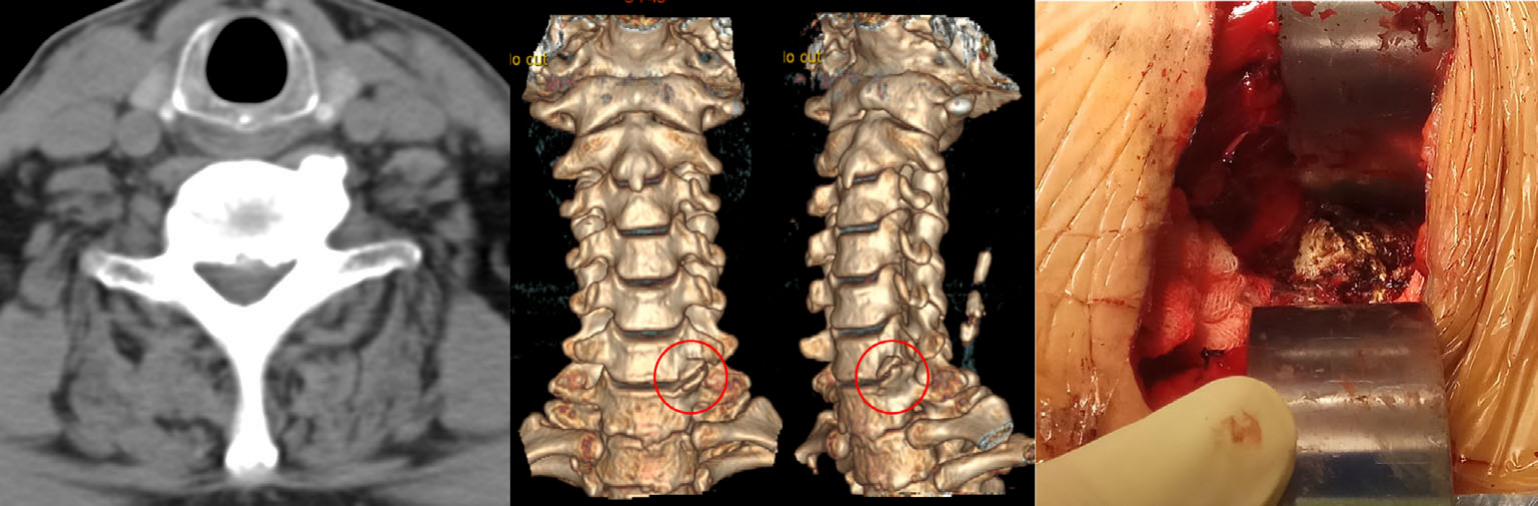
Cervical CT scans demonstrate the Luschka's joint osteophyte in patient's degenerative cervical vertebrae (red circle); the exposure and resection of Luschka's joint osteophytes during the anterior cervical decompressive surgery.
